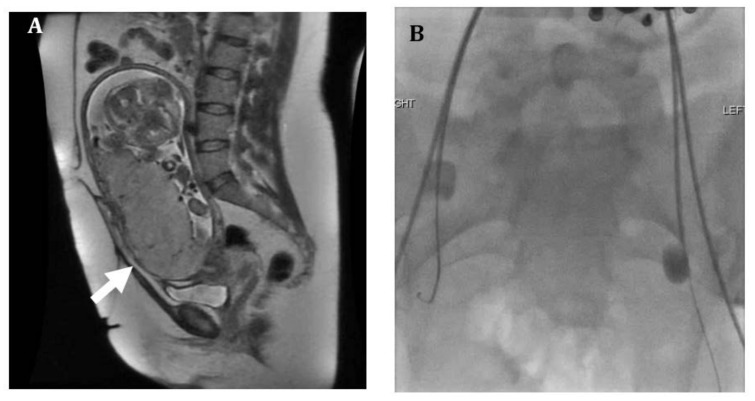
Thirty-three-year-old female with placenta accreta. (A) Sagittal SSFSE MR image shows a thickened placenta with abnormal lobulated contour and loss of myometrial thickness in the lower uterine segment (arrow), suggestive of placenta accreta. (B) Prophylactic bilateral internal iliac artery occlusion balloons were placed prior to hysterectomy. Patient underwent cesarean hysterectomy at 33 weeks with delivery of a healthy newborn. Pathology showed superficial attachment of the placenta to the uterine wall, consistent with placenta accreta.
